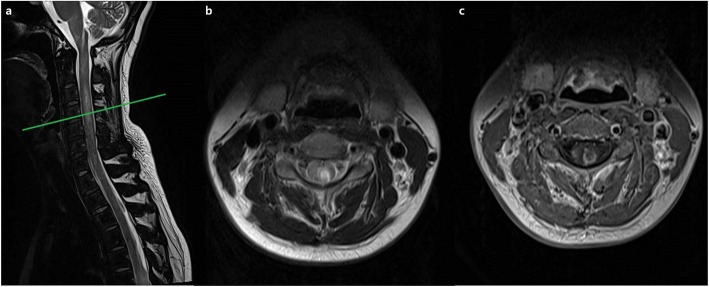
a, b, c Spine MRI taken at the second neurologic attack. (a) Sagittal spine MRI showing longitudinal extensive transverse myelitis from the medulla oblongata to the thoracic spine level on the T2-weighted image. Axial T2-weighted MRI (b) and T1-weighted MRI with contrast enhancement (c) at C4 (mark by the green line)
